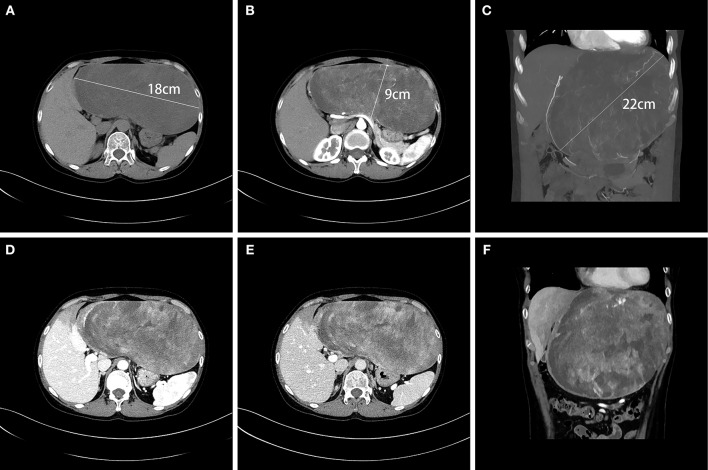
CT showed a large, regular, hypodense 22 × 18 × 9 cm mass in the left lateral lobe of the liver (A–C). Blood vessels were observed in the tumor in the arterial phase (B,C), and the tumor was progressively unevenly enhanced in the portal vein phase (D) and in the delayed phase (E). The liver tissue adjacent to the tumor was weakly enhanced (F).
